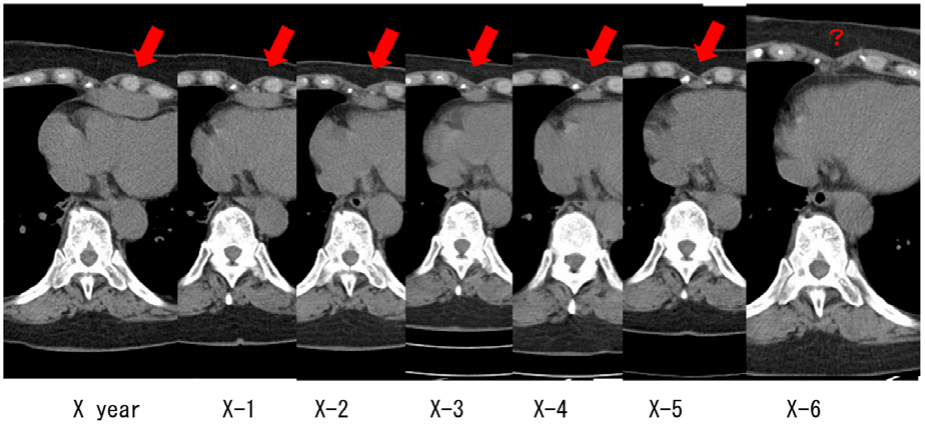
Chest computed tomography image showing a mediastinal tumour over 6 years
